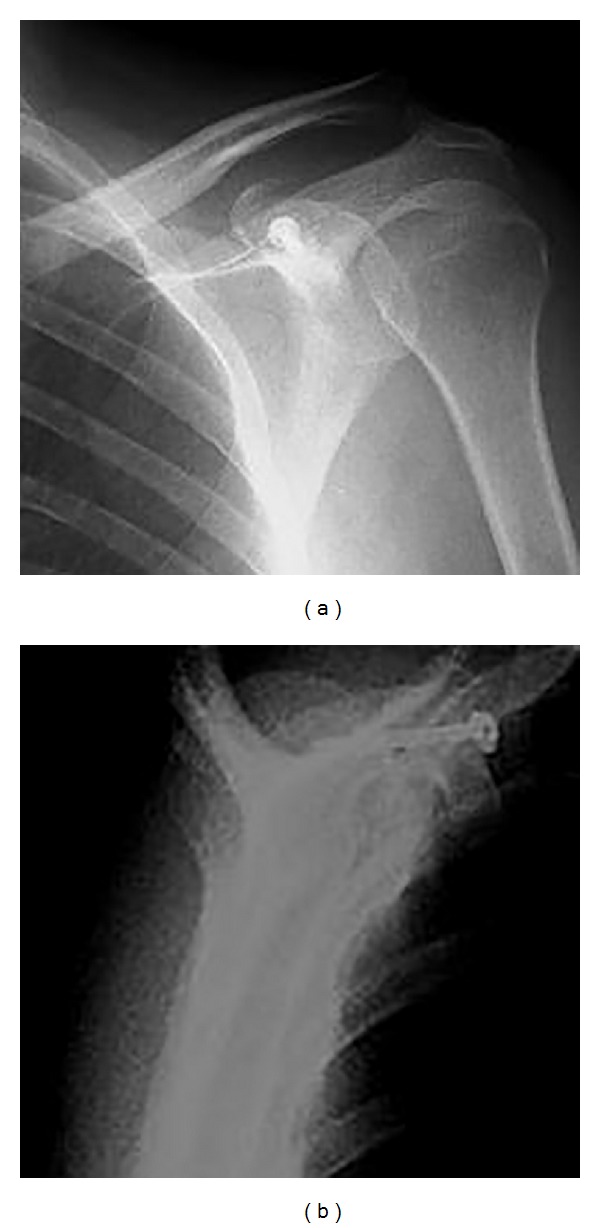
Postoperative 6th week X-ray images (fracture fixed bicortical).
